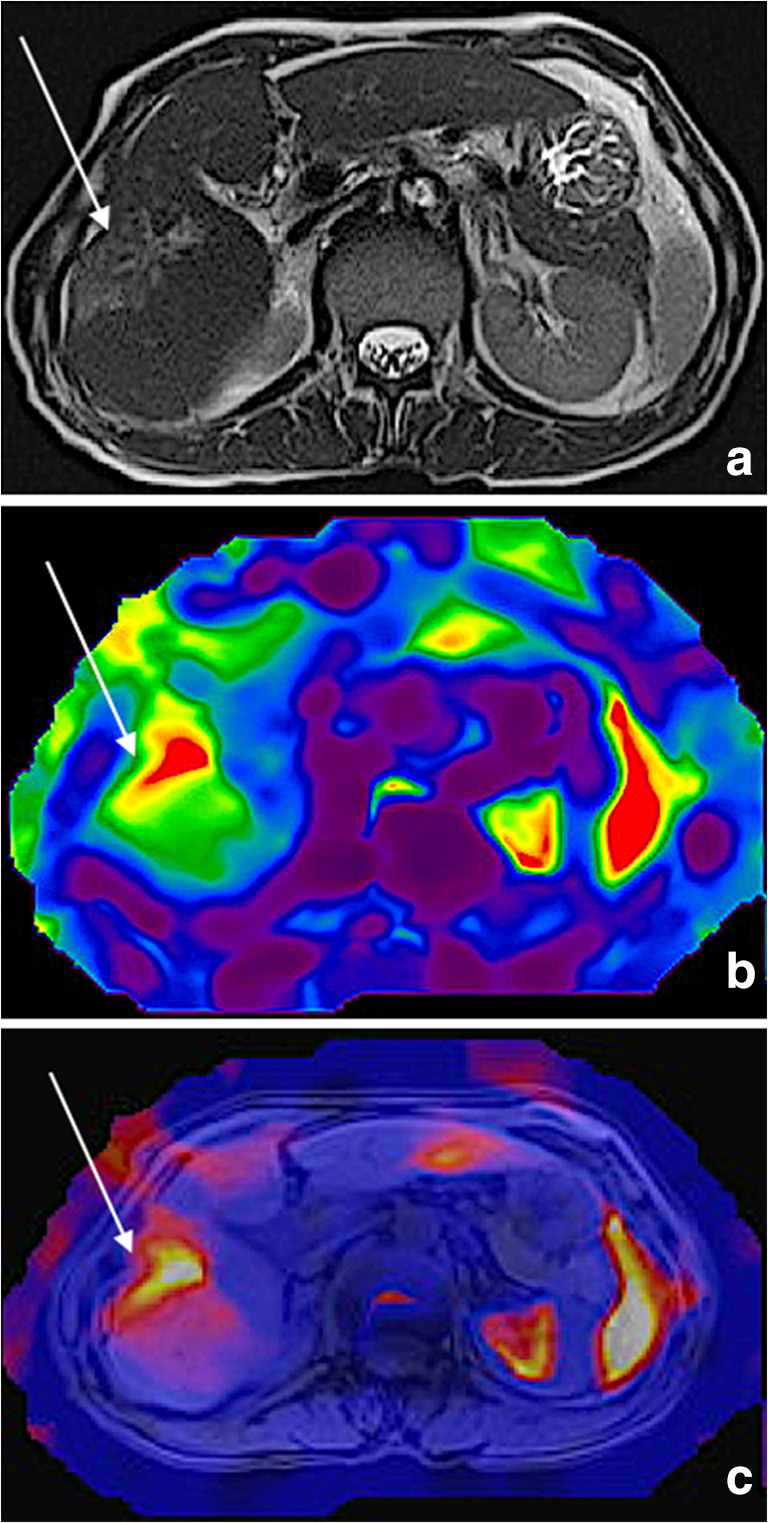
A 64-old-male patient with colorectal liver metastasis in the right hepatic lobe. The metastasis is visualized in both images (arrows). The native MRI series (a) were matched with MRE data and merged to a tumor-stiffness 3D fusion for parameter analysis (b, c)
